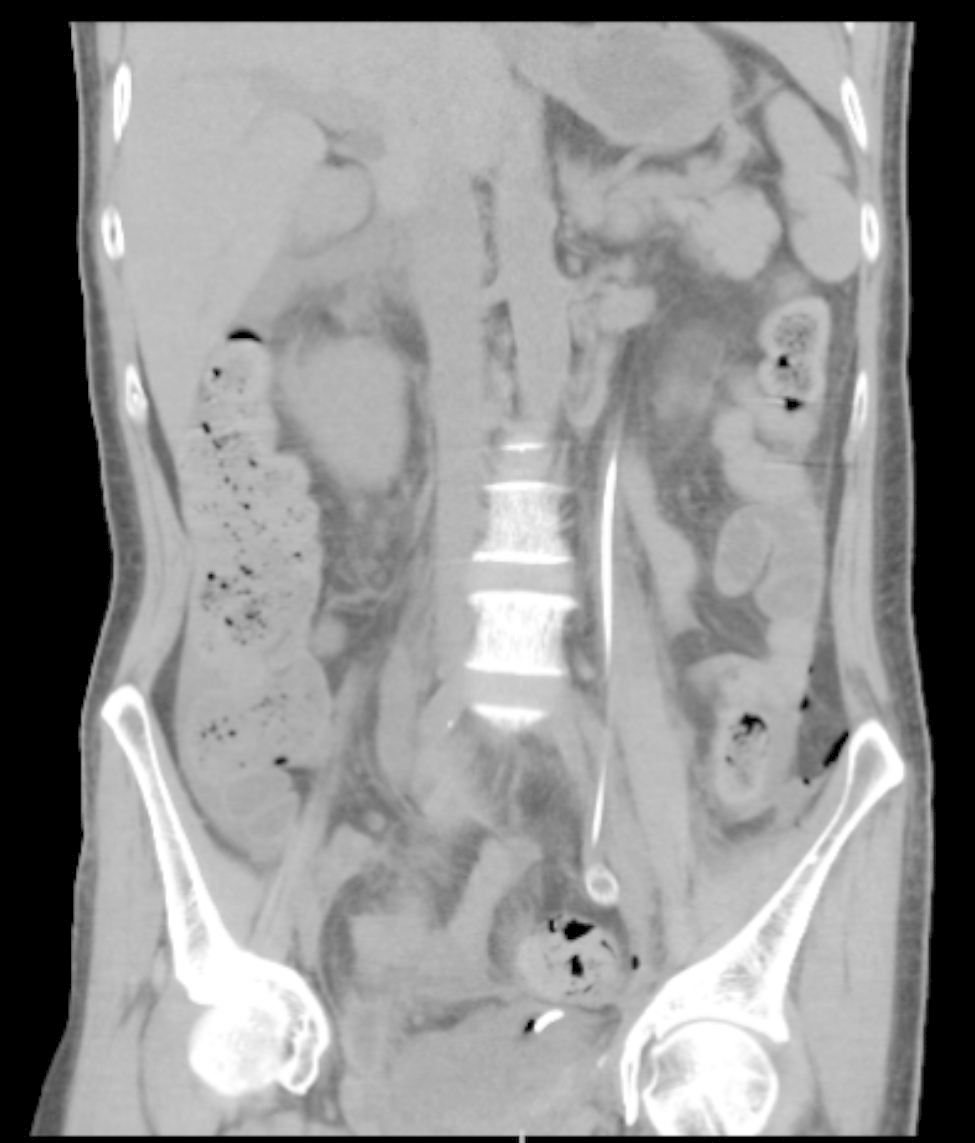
CT reconstruction suggests that the tumor progressed and induced bilateral hydronephrosis, only the left ureteral stent can be placed
